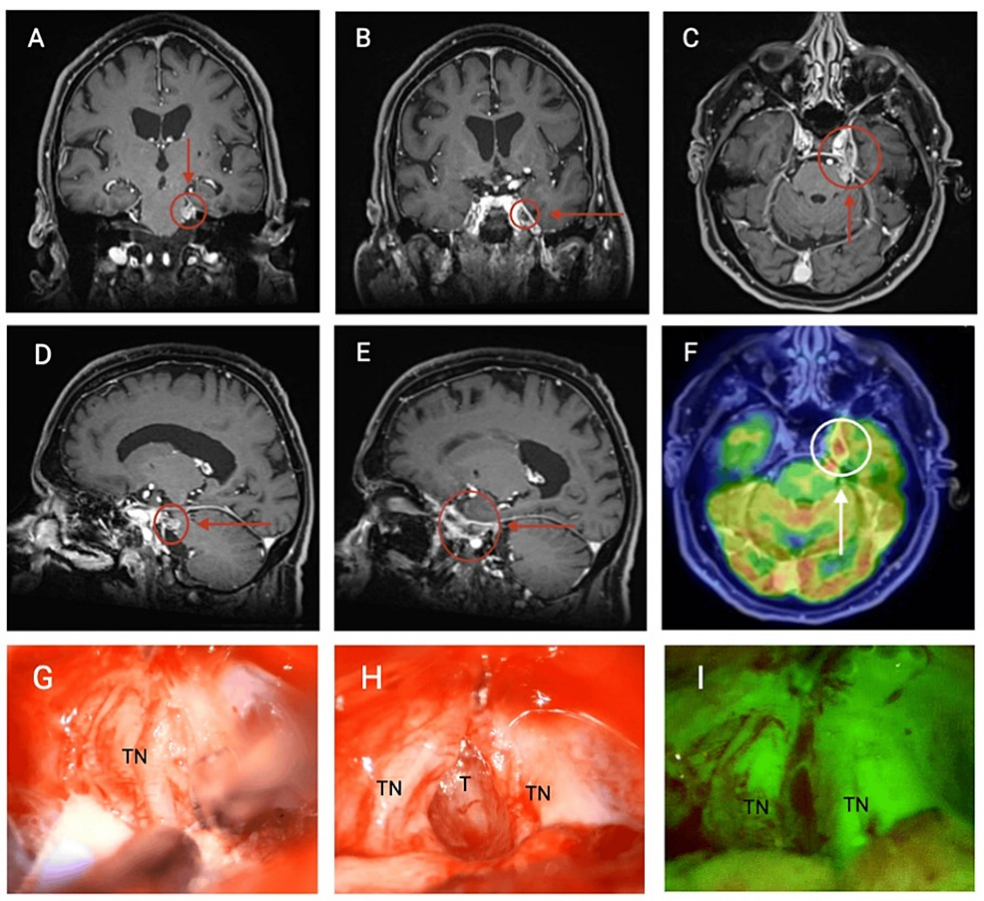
Trigeminal nerve invasion. MRI with gadolinium in coronal (A, B), axial (C), and sagittal (D, E) planes showing an inflammatory process that involves the trajectory of the left trigeminal nerve with extension towards the ipsilateral cavernous sinus, which shows continuity through the superior orbital fissure through the ophthalmic branch of the trigeminal cranial nerve (V1), oculomotor nerve (third cranial nerve), and trochlear nerve (fourth cranial nerve) as well as the mandibular and maxillary branch (V2, V3) in the trajectory of foramen ovale and rotundum. 18-FDG PET/CT scan in the axial plane (F) showed a hypermetabolic lesion in the left cavernous sinus involving the left trigeminal trajectory (6.9 SUVmax). A retrosigmoid craniotomy showed the left trigeminal nerve (G) with perineural tumor invasion positive to fluorescein (I).  The nerve was dissected and opened to take a core central biopsy of the tumor (H). PET: positron emission tomography
